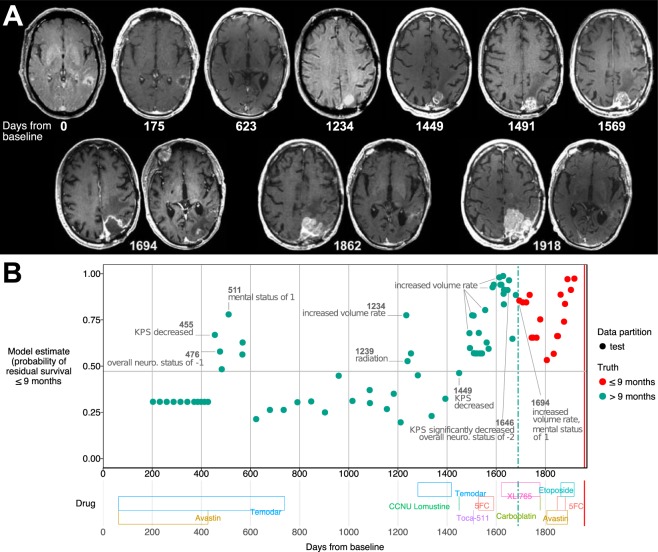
An example of a white male initially diagnosed in his 60′s. (A) MR imaging showing tumor at baseline, regrowth, and remote recurrence near end of life. (B) The 9-month residual survival model’s estimates given patient covariates and temporal patterns observed at each clinical visit. Gray horizontal line is a threshold (see Supplemental Methods) used for classifying residual survival from the predicted probabilities. The vertical green dash line is 9 months (270 days) from the vertical red line (day of death).
